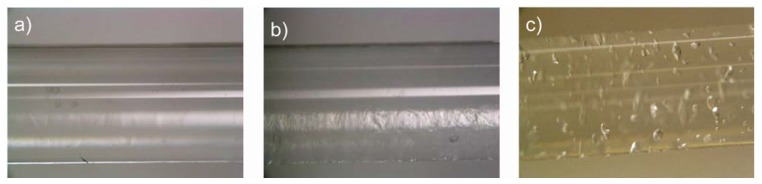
Representative photographs of hydrogels swollen in 2 mm diameter glass capillaries with deionized water. (a) Cabloc CT, (b) Produkt Z 1069, and (d) Favor pac 230. White lines on the tubes are reflections on the glass from the lamp used to illuminate the samples.
